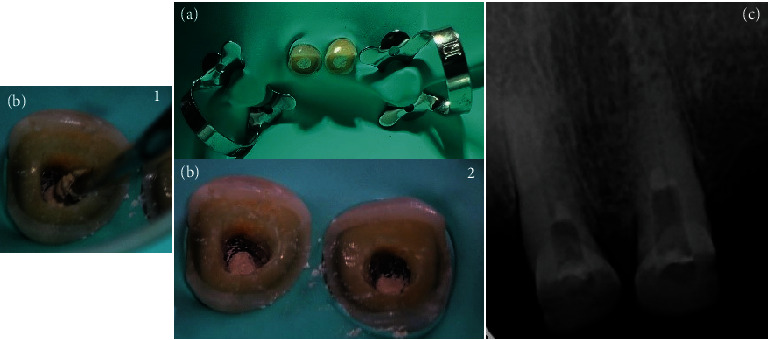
(a) Isolation of both teeth with rubber dam; ((b) 1 and 2) perforation sealing; and (c) postoperative radiograph with perforation sealing.
